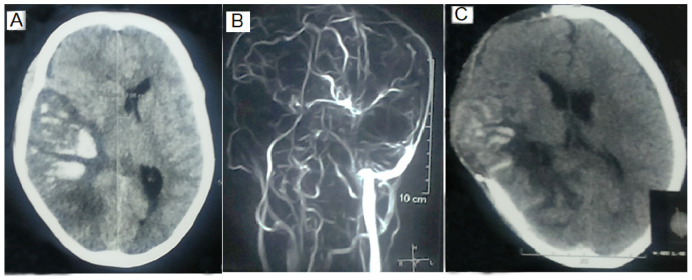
CT scan and MR venography of a patient with cerebral venous thrombosis showing hemorrhagic infarction in the parieto-occipital area with effacement of sulci and ventricle and midline shift (A). On MR venography, there was thrombosis of both the superficial and deep systems (B). The patient underwent hemicraniectomy on the third day of hospitalization, and the postoperative CT scan revealed improvement in mass effect as evidenced by the reappearance of the lateral ventricle and reduced midline shift (C).
